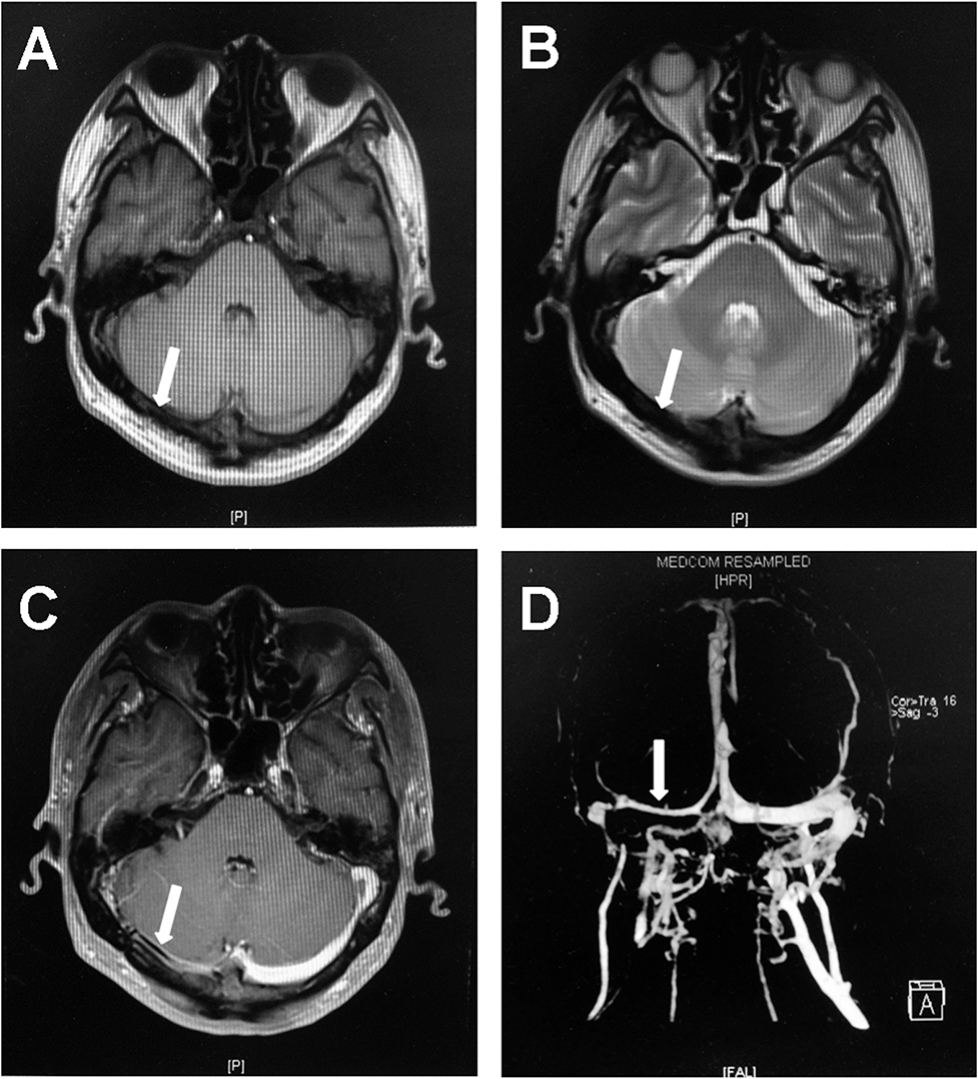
Transverse sinus stenosis with double-track sign. A 37-year old female patient with right transverse sinus stenosis. Hypointense on T1WI (A) and T2WI (B) demonstrated a chronic stage of thrombus, and double-track sign (arrow) was detected on axial Gd-enhanced T1WI (C). Transverse sinus stenosis was confirmed on MRV which showed a linear signal of blood flow in the right transverse sinus (D). (Time from MRI to MRV was 4.5 hours).
